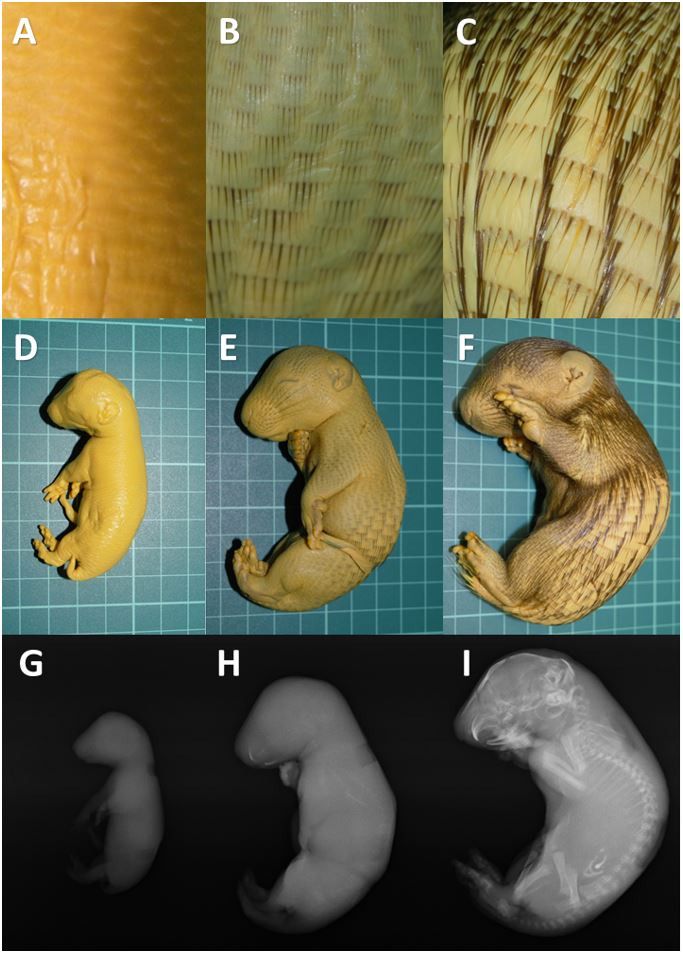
Hair pattern, fetus morphology, and x-ray imaging of three *Hystrix javanica* fetuses.
